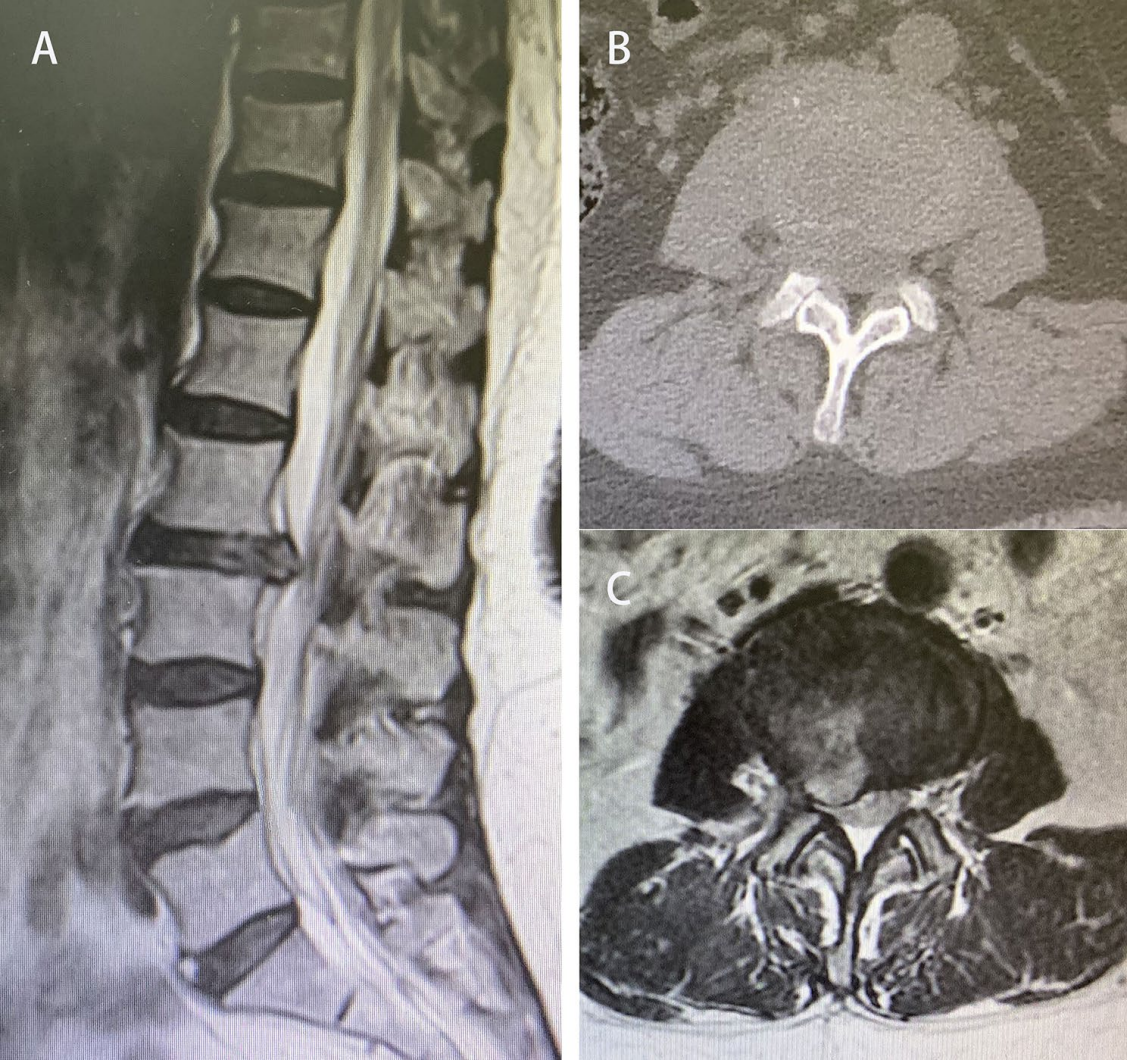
Presurgical MRI showing L2-L3 disc herniation. (A) Sagittal view. (B and C) Axial views of L2-L3 (B, CT; C, MRI).
